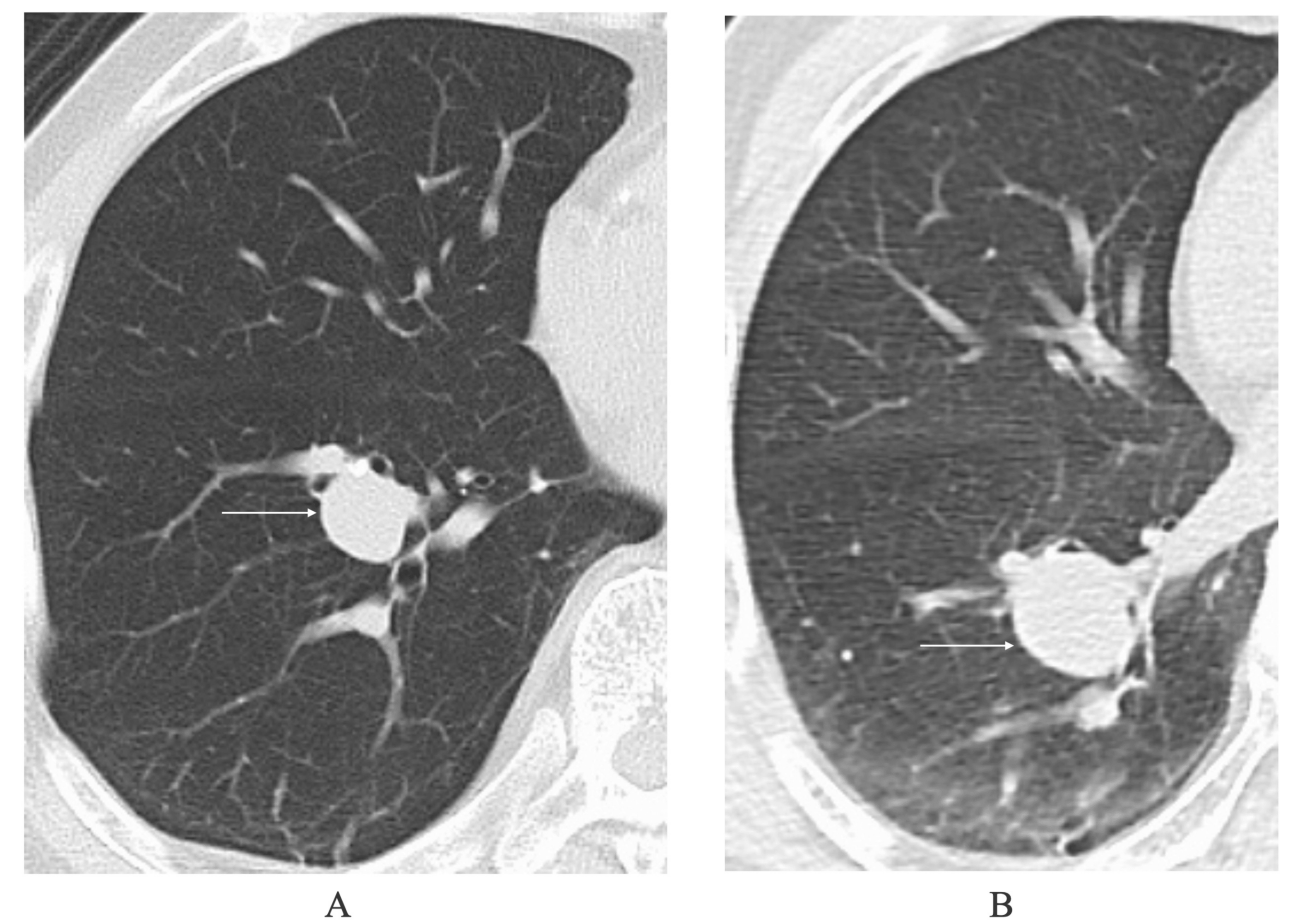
Computed tomography (CT) findings A. CT showed a pulmonary nodule (arrow) in close contact with the inferior pulmonary vein. B. One year observation caused growth of the pulmonary nodule (arrow).
